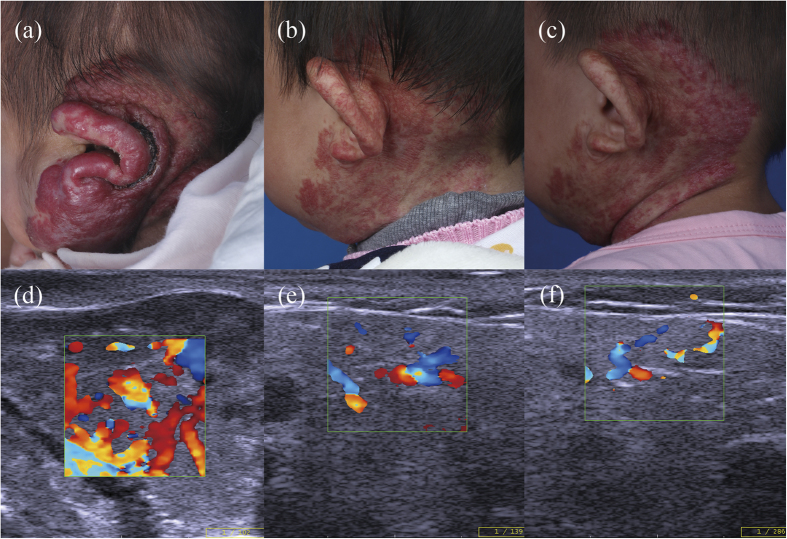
A typical case involving partial regression. (a) Before treatment, a 3-month-old girl presented with a large mixed hemangioma in her left parotid region. (b) After 15 months of propranolol treatment, maximal reduction was achieved for more than three months. Propranolol was stopped gradually over 4 weeks. (c) Six months after propranolol was stopped, the residual lesion remained stable. (d,e,f) Corresponding color Doppler ultrasound images of (a,b and c).
